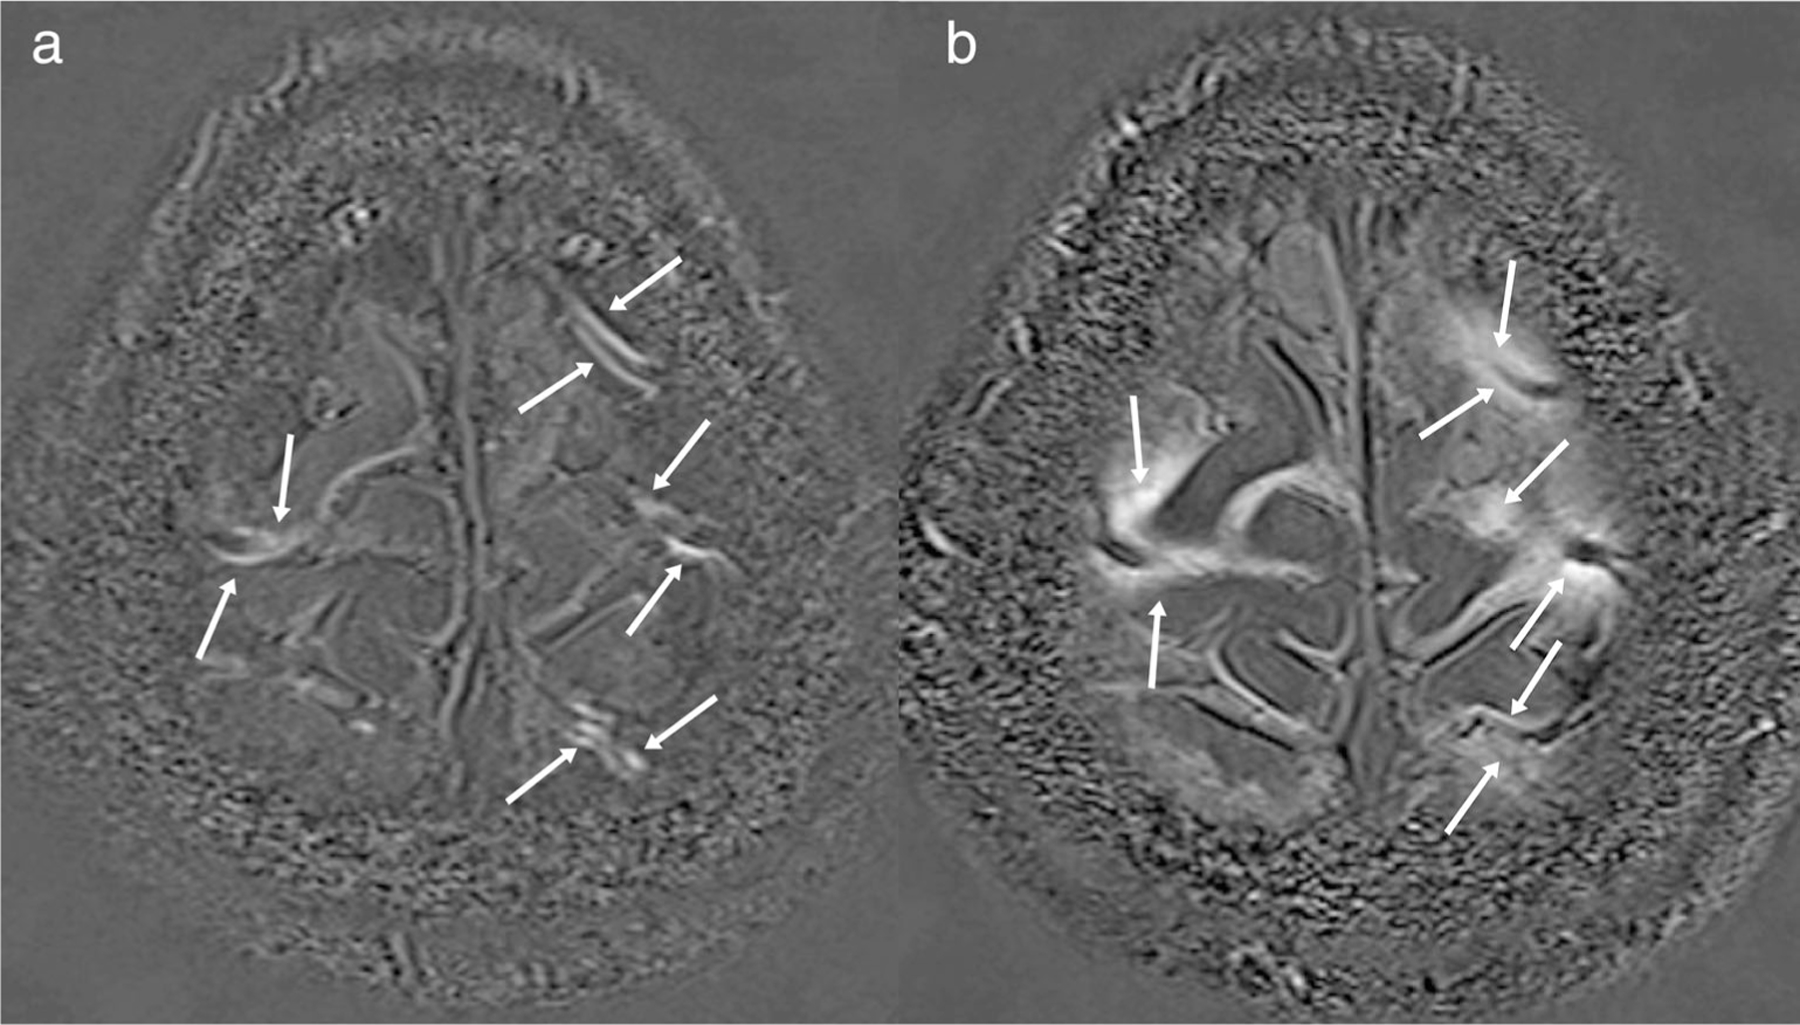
Intravenous (IV) enhanced delayed MRI: Subtracted 3D-real IR images obtained in a 60s male patient with a suspicion of endolymphatic hydrops. (a) Subtraction of pre-contrast 3D-real IR image from the 3D-real IR image obtained 5 minutes after IV administration of single dose gadolinium-based contrast agent (IV-GBCA). Linear structures with contrast enhancement are visualized along the cortical veins (arrows). These linear structures are speculated to be the subpial perivenous drainage routes. (b) Subtraction of pre-contrast 3D-real IR image from the 3D-real IR image obtained 4 hours after IV-GBCA. Contrast enhancement spread to SAS around cortical veins (arrows). The degree of the GBCA leakage to CSF has been reported to accelerate with aging.
